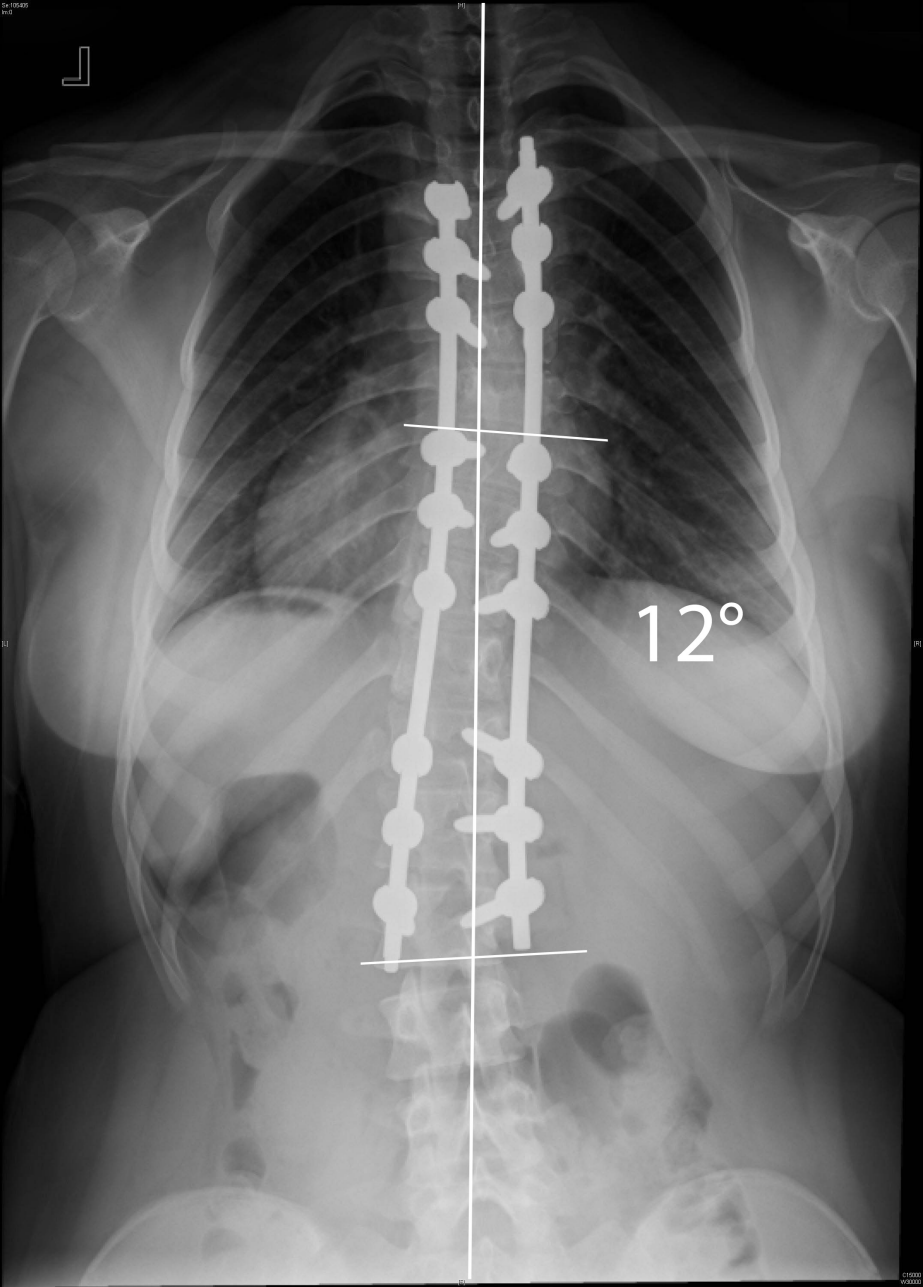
"Patient 1 - 2-year postoperative anteroposterior and lateral radiographs." 2-year postoperative correction anteroposterior and lateral X-rays of the patient in Figure 9. A 3-3-3 pattern of instrumentation was utilized. The patient shows good correction, is well balanced in the coronal plane, the shoulders are level, and the dorsolumbar kyphosis has been restored to normal.
